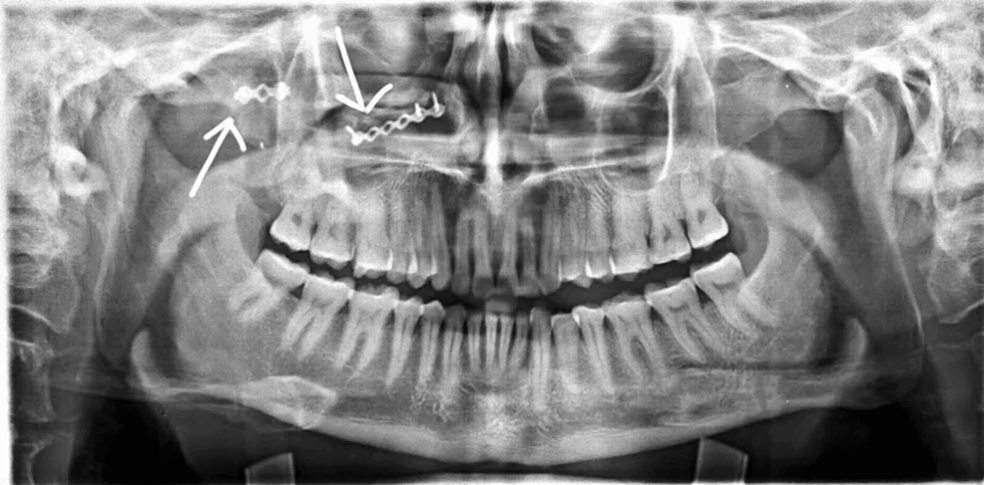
Postoperative radiograph showing fixation.
